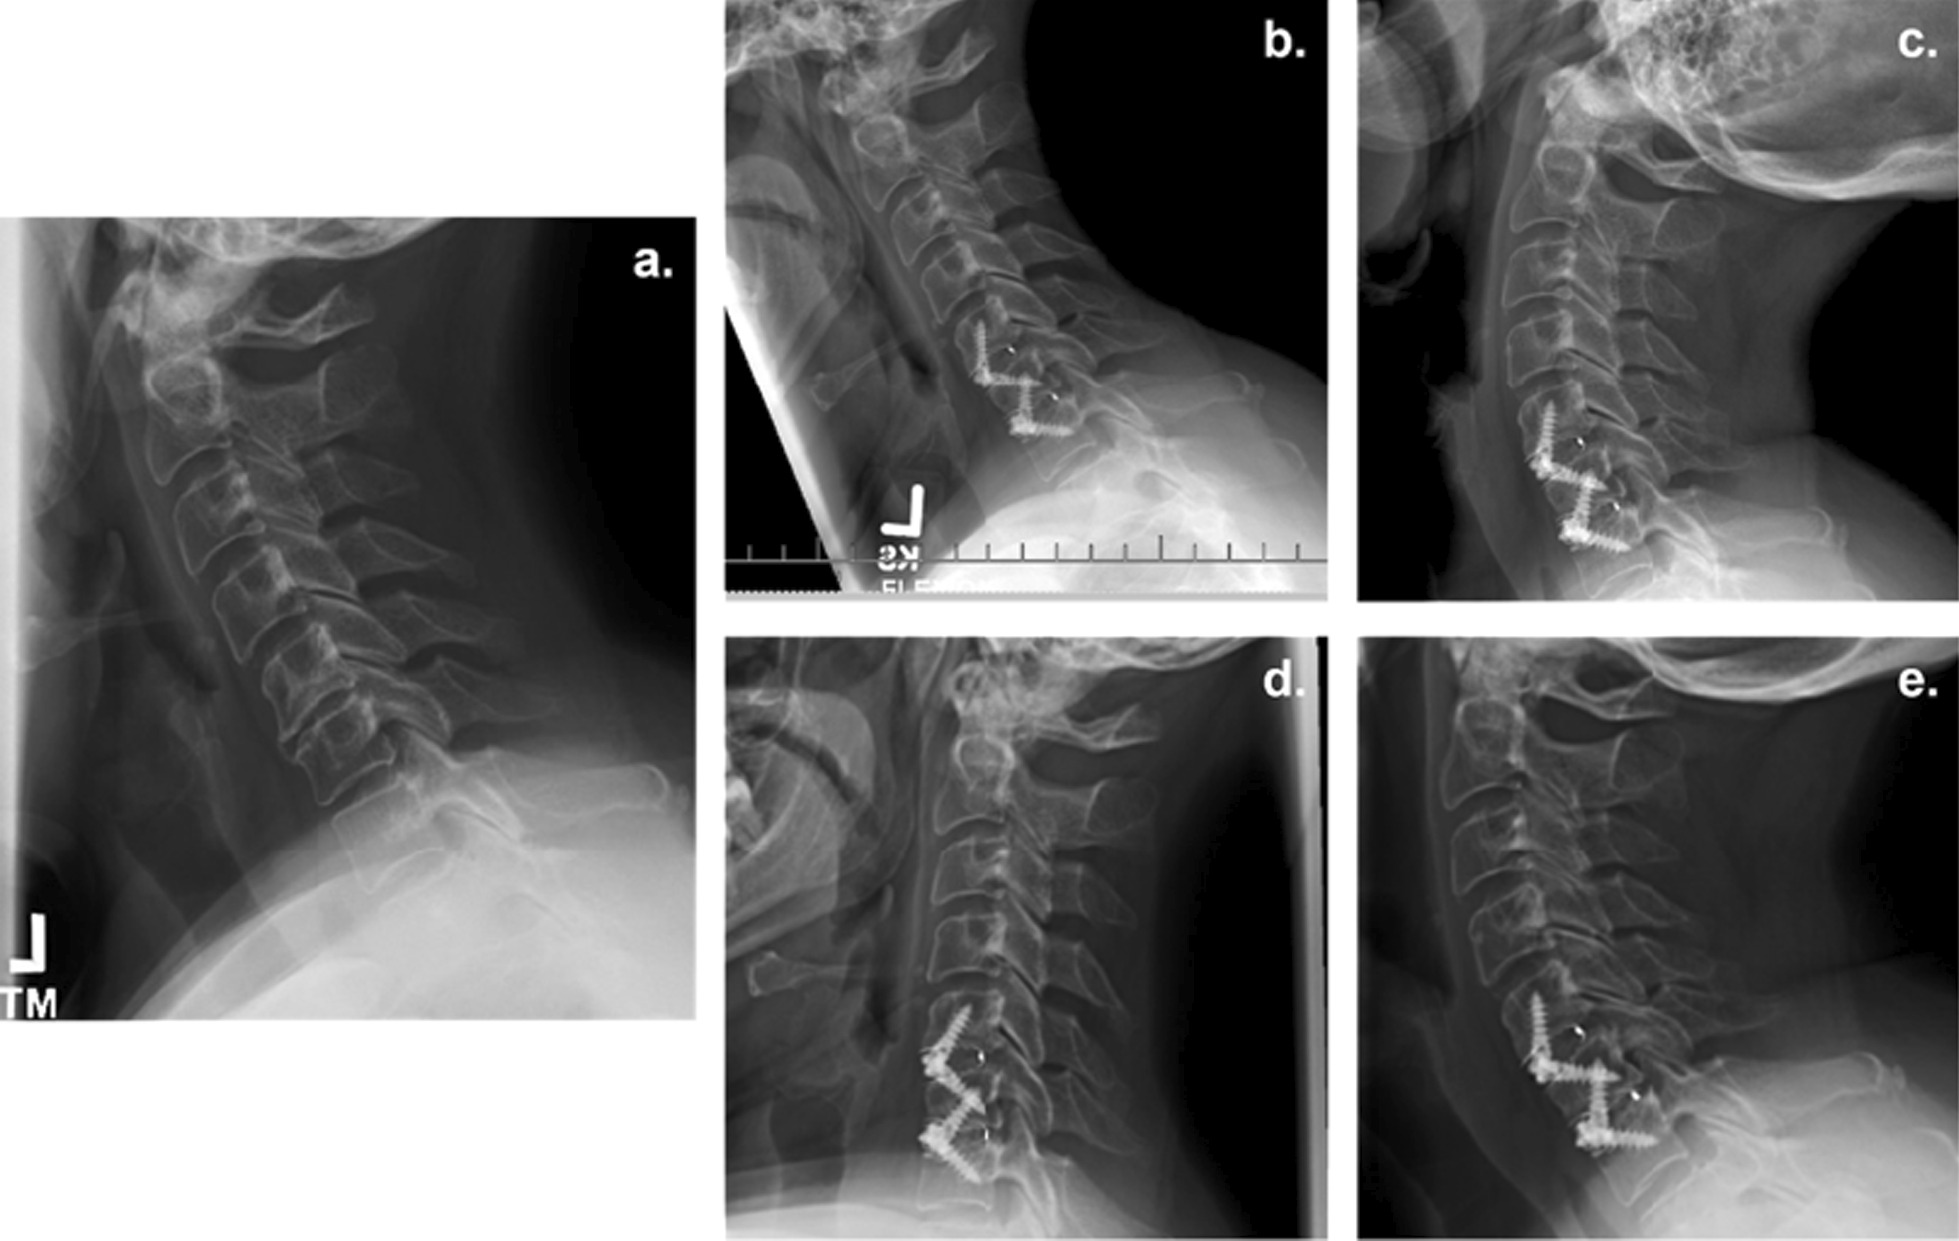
Pre- and post-operative radiographs of a 2-level ACDF using ViBone on a 58-year-old obese, current smoker, type II diabetic female at C5–C6 and C6–C7. a Pre-operative lateral radiograph b six-month flexion radiograph c six-month extension radiograph d Twelve-month flexion radiograph e Twelve-month extension radiograph
